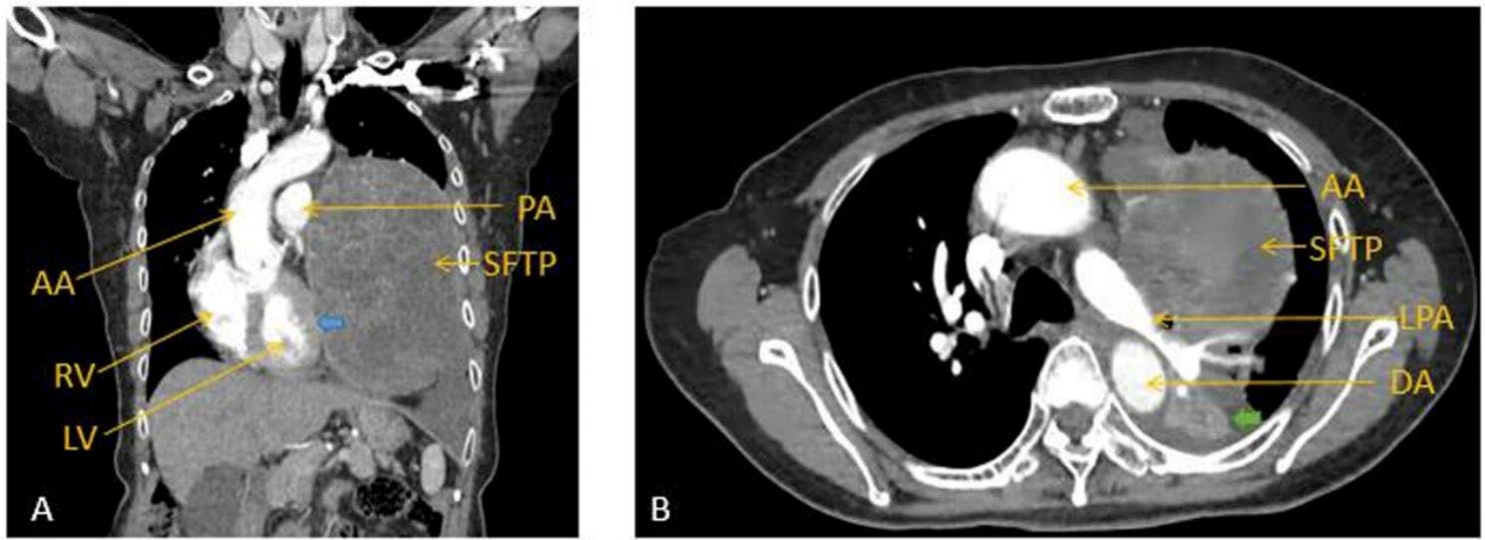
Chest contrast-enhanced computed tomography (CT) demonstrating massive opacification in the left hemithorax. A. Coronal image revealed the solitary fibrous tumor of the pleura (SFTP) occupying the majority of left hemithorax. Left ventricle (LV) was compressed by the tumor. The boundary between the pericardium outside the left ventricle and the tumor is not clear (blue arrow). Right ventricle (RV), ascending arota (AA) and pulmonary artery (PA) was pushed to the right. B. Axial image showed left bronchial obstruction and left lower lobe atelectasis because of the compression of SFTP (green arrow). Left pulmonary artery (LPA) was compressed severely. Ascending arota (AA) and descending arota (DA) was compressed as well
